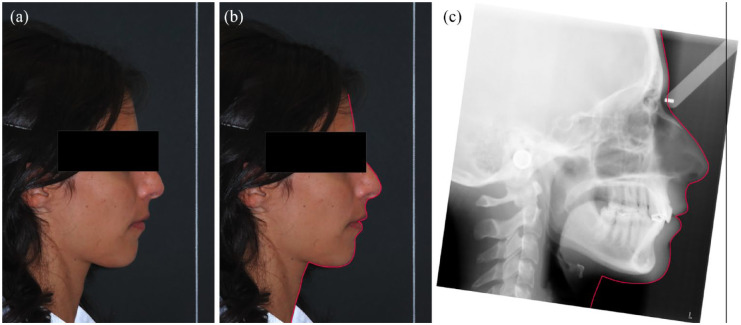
(a) Profile photograph in NHP with TV line, (b) soft tissue profile traced on the profile photograph and (c) cephalometric radiograph rotated accordingly to NHP. NHP, natural head position; TV, true vertical.
